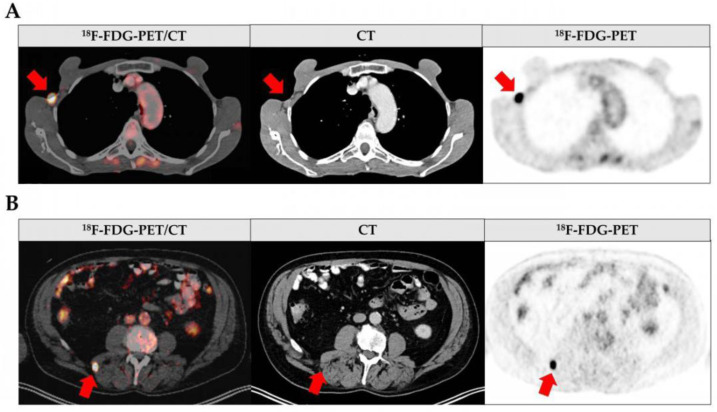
Images from ^18^F-FDG-PET/CT at melanoma diagnosis. Fused ^18^F-FDG-PET/CT (left), CT (middle) and ^18^F-FDG-PET (right) scans. Metastases are indicated by red arrows. (A) shows an axillary lymphatic metastasis in a 55-year-old patient and (B) depicts a metastasis in the autochthonous back muscles in a 66-year-old patient.
